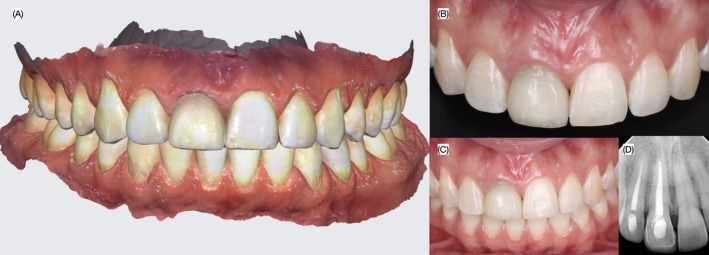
Intra‐oral evaluation. (A) Intra‐oral scan, (B) view of anterior teeth, (C) in occlusion and (D) radiograph.
